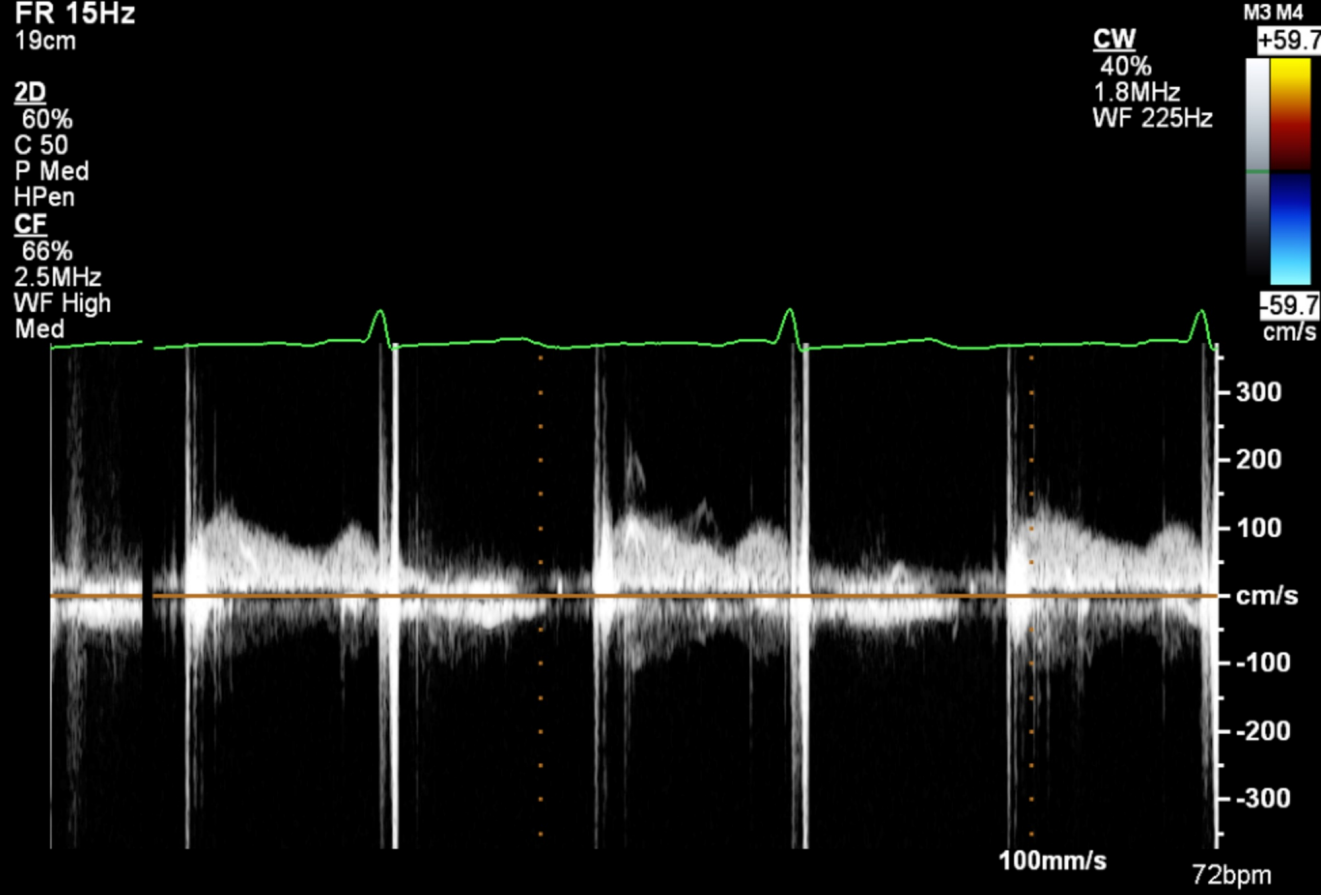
Transthoracic echocardiography doppler measurements showing normal mean pressure gradient across mechanical mitral valve prior to pregnancy
